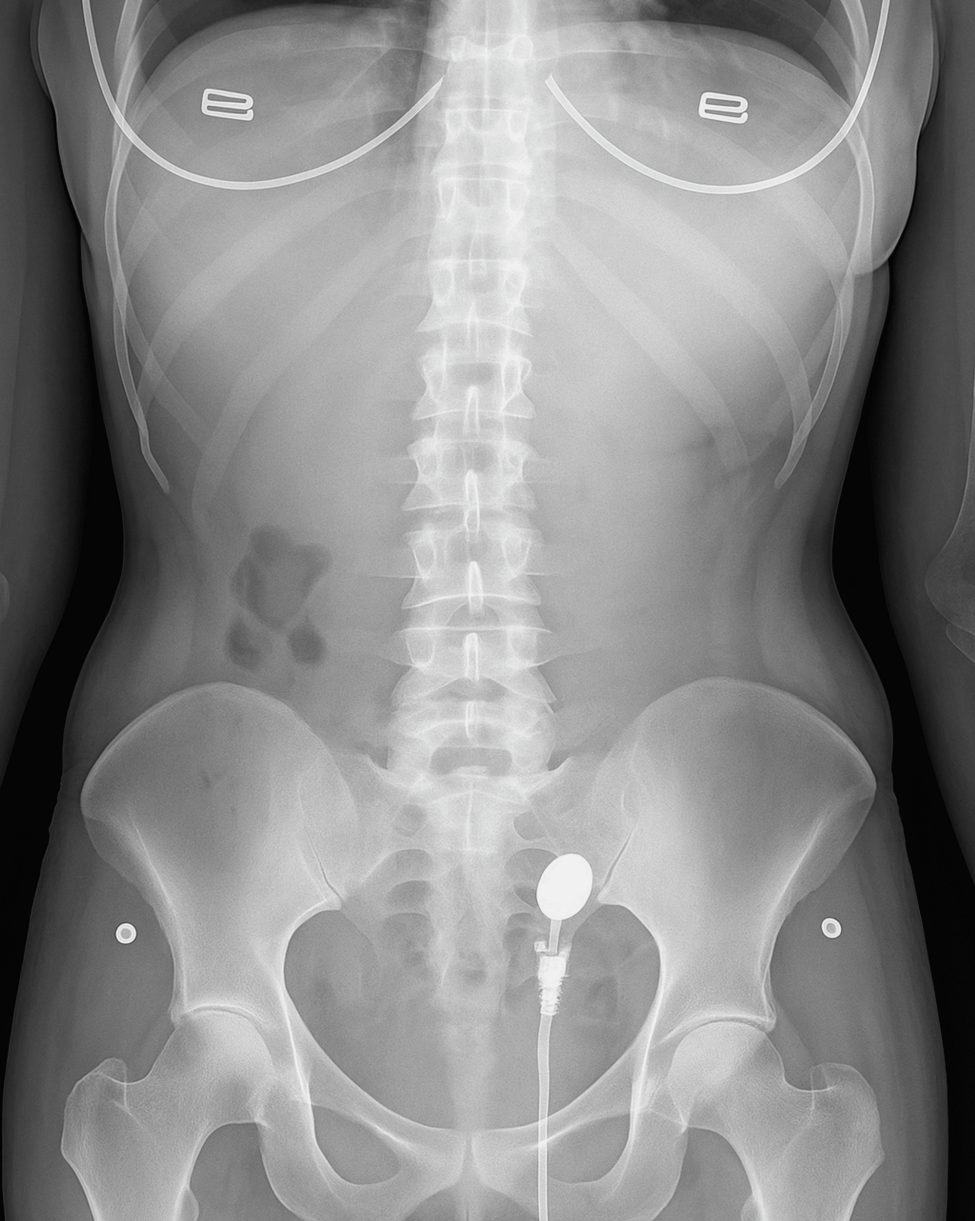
plain abdomen X-ray of the patient
